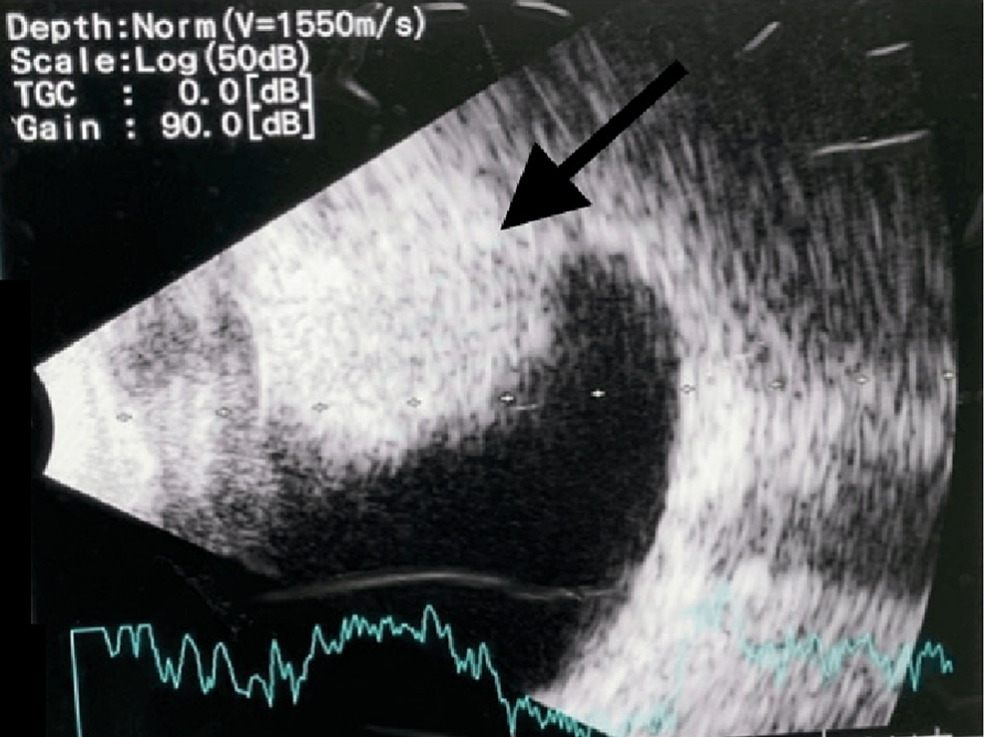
B-scan ultrasonography of the left eye at initial presentation, showing a well-circumscribed homogenous mass (black arrow)
